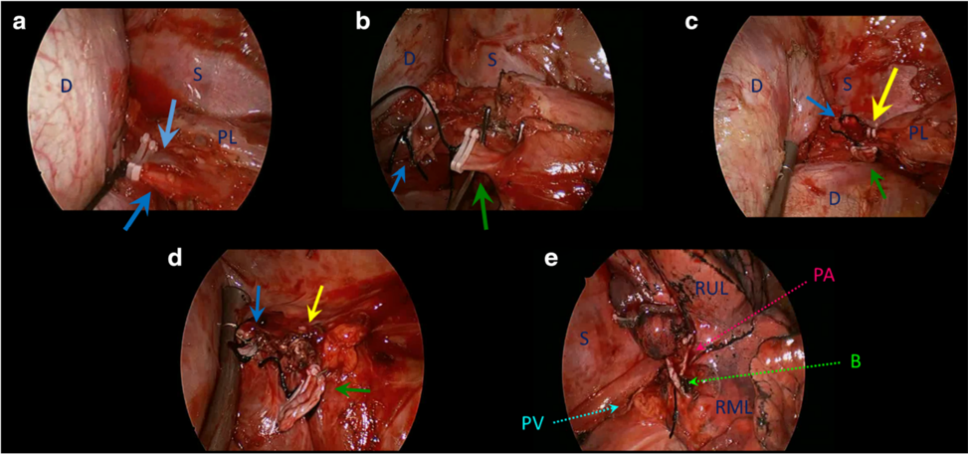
Intra-operative views (legend: D = diaphragm; S = spine; PL = pulmonary ligament; RUL = right upper lobe; RML = right middle lobe). a The first aberrant feeding vessel entered the chest by penetration through the posterior diaphragm. It promptly divides into two branches once in the chest (light and dark blue arrows respectively). This vessel has been secured proximally with silk ligature and double vascular clips prior to staple-division. b The second aberrant feeding vessel (green arrow) also entered the chest by penetration through the posterior diaphragm (blue arrow = stump of first aberrant vessel). This second vessel has been secured proximally with silk ligature and double vascular clips prior to staple-division. This view gives some impression of the extensive adhesiolysis that was required around the right lung base, diaphragm and spinal area in order to expose these aberrant feeding vessels. c The third aberrant feeding vessel (yellow arrow) entered the chest along the pulmonary ligament (blue arrow = stump of first aberrant vessel; green arrow = stump of second aberrant vessel). This small third vessel has been secured proximally with double vascular clips prior to division using an ultrasonic energy device. Notice that the diaphragm has been retracted using the suction to expose the vessels. d View of the stumps of all 3 aberrant feeding vessels following division (blue arrow = first vessel; green arrow = second vessel; yellow arrow = third vessel). e View of the hilum following Uniportal right lower lobectomy, showing the stapled stumps of the inferior pulmonary vein (PV), right lower lobar pulmonary artery (PA), and right lower lobar bronchus (B)
